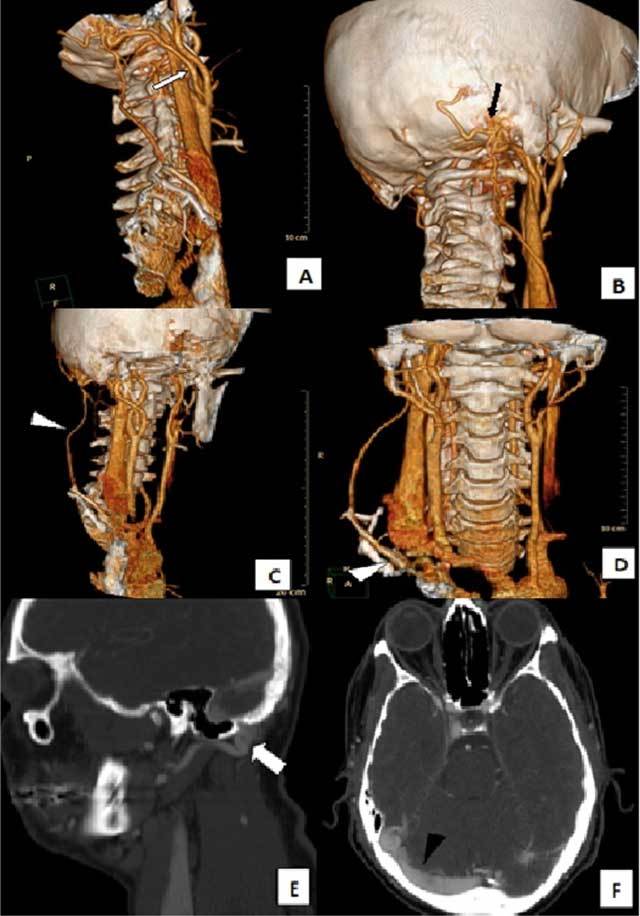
On CT angiography, (a) an enlarged right occipital artery was identified as the feeding artery of the DAVF (white arrow) and the (b) large, tortuous vascular structures were seen in the right mastoid region (black arrow). The (c–d) auxiliary/alternative venous drainage path to the right brachiocephalic vein (white arrowhead), (e) the location of the fistula in the suboccipital-mastoid region and enlarged vascular structures (thick white arrow), and (f) the typical drainage of DAVF to the right transverse-sigmoid sinus were determined.
